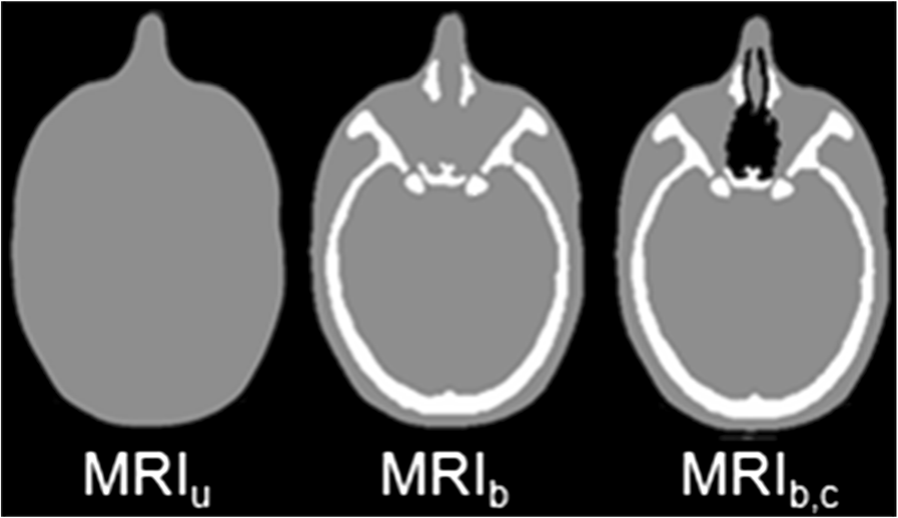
The different density corrected MRIs for a HN patient. Left: A homogeneous density assigned MRI. Middle: A bulk density assigned MRI. Right: A bulk density assigned MRI including air cavities.
